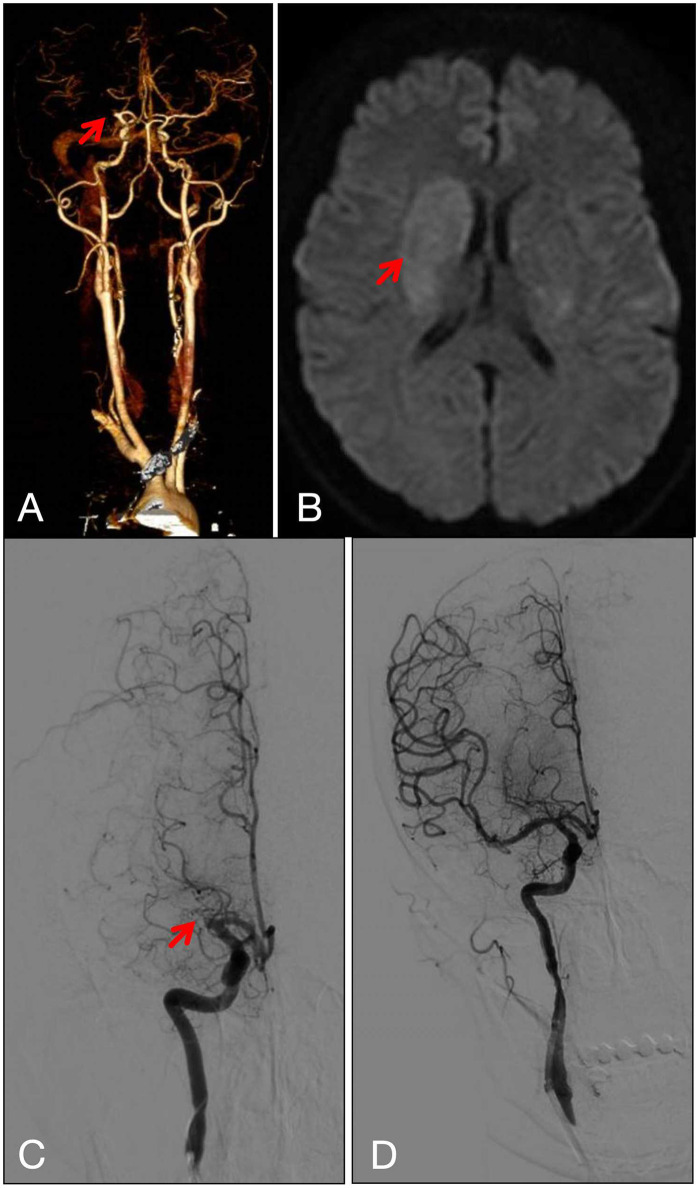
(A) CTA showing occlusion of the right MCA M1 segment (red arrow), (B) MRI-DWI showing acute infarction in the right basal ganglia (red arrow). Angiography showing (C) occlusion of the M1 segment of the left middle cerebral artery (red arrow) and (D) successful revascularization.
